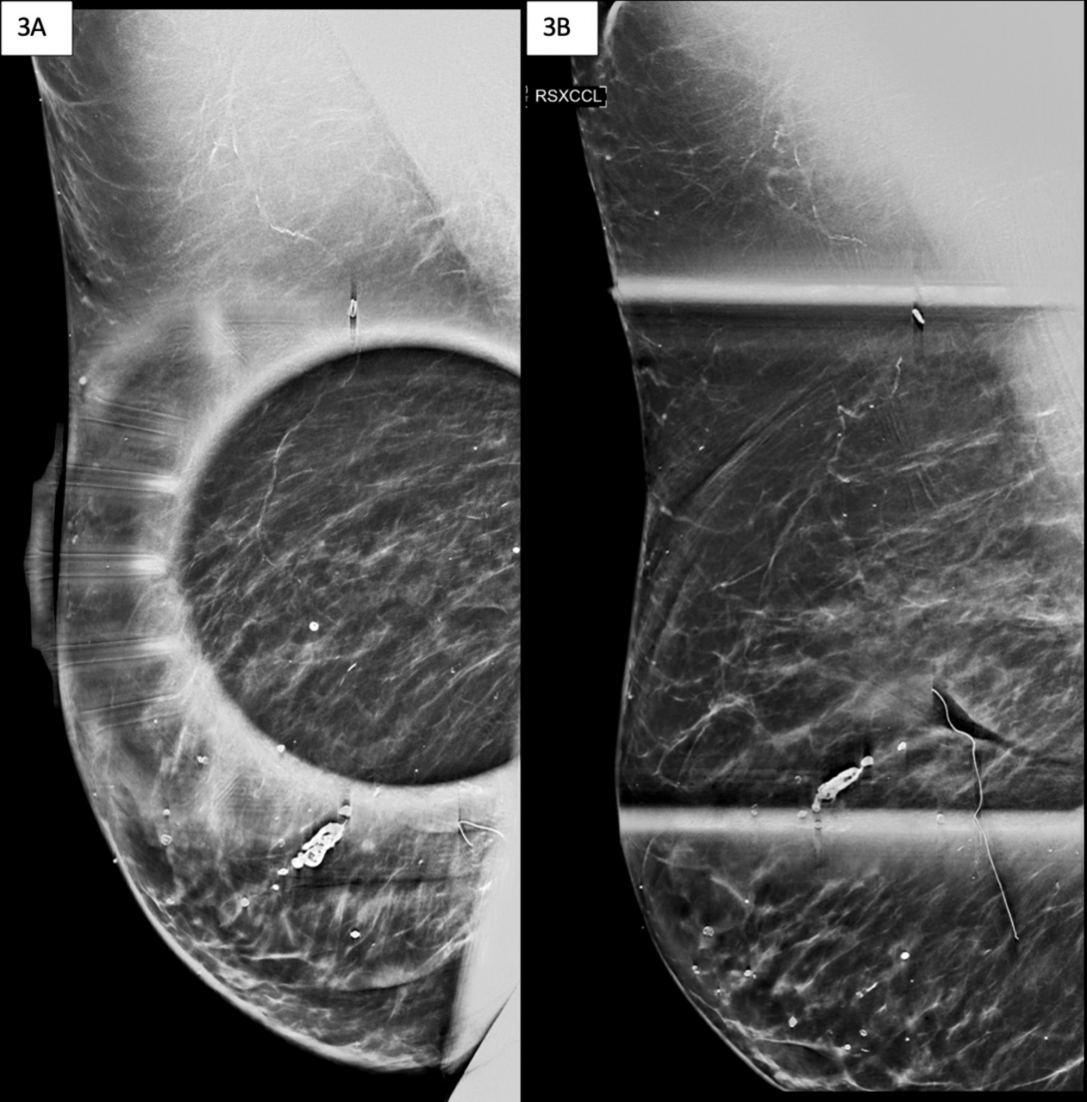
Images of diagnostic mammogram Image A details right breast spot compression in the medial lateral oblique view (RSMLO) of the lumpectomy bed. Image B details right breast spot compression exaggerated in the craniocaudal view (RSXCCL) of the lumpectomy bed. There are postlumpectomy changes with no suspicious masses, calcifications, or abnormal findings. Again, the abnormal CT finding was far posterior and thus not included in the field of view on mammogram.
